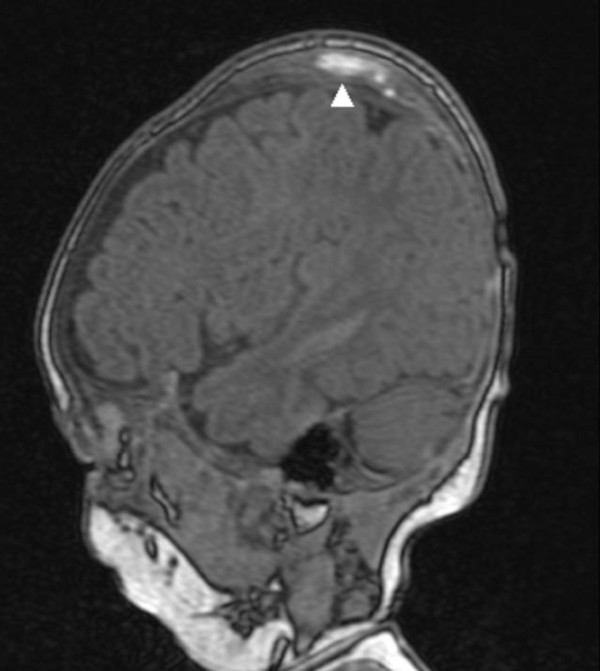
Sagittal T1-weighted MR image showed significant expansile lesion associated with a dense zone of calcification within the mass-arrow (fig 4).
